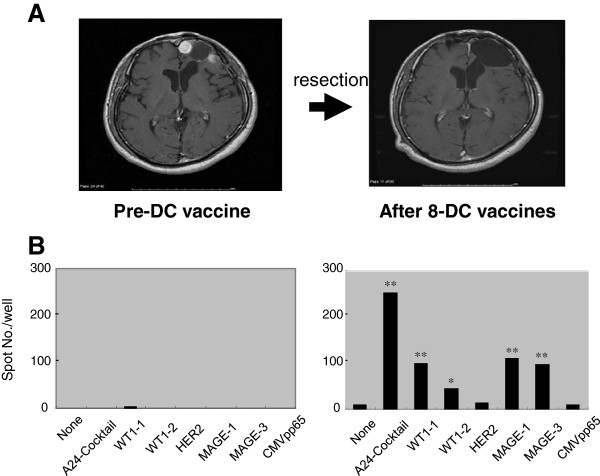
Effect of DC vaccines on tumor progression and CTL induction in patient 2. A; Maintenance of progression-free status for as long as 34 months by DC vaccines after surgical resection as revealed by MRI. B; Remarkable CTL activity after 8 DC vaccines as revealed by ELISPOT assay. The spot number per well of peptide-stimulated CTLs was compared to that of a negative well without peptide using Student’s paired two-tailed *t-*test. Statistically significant compared to no peptide, *; *P* < 0.05, ; *P* < 0.01. None; no peptide.
